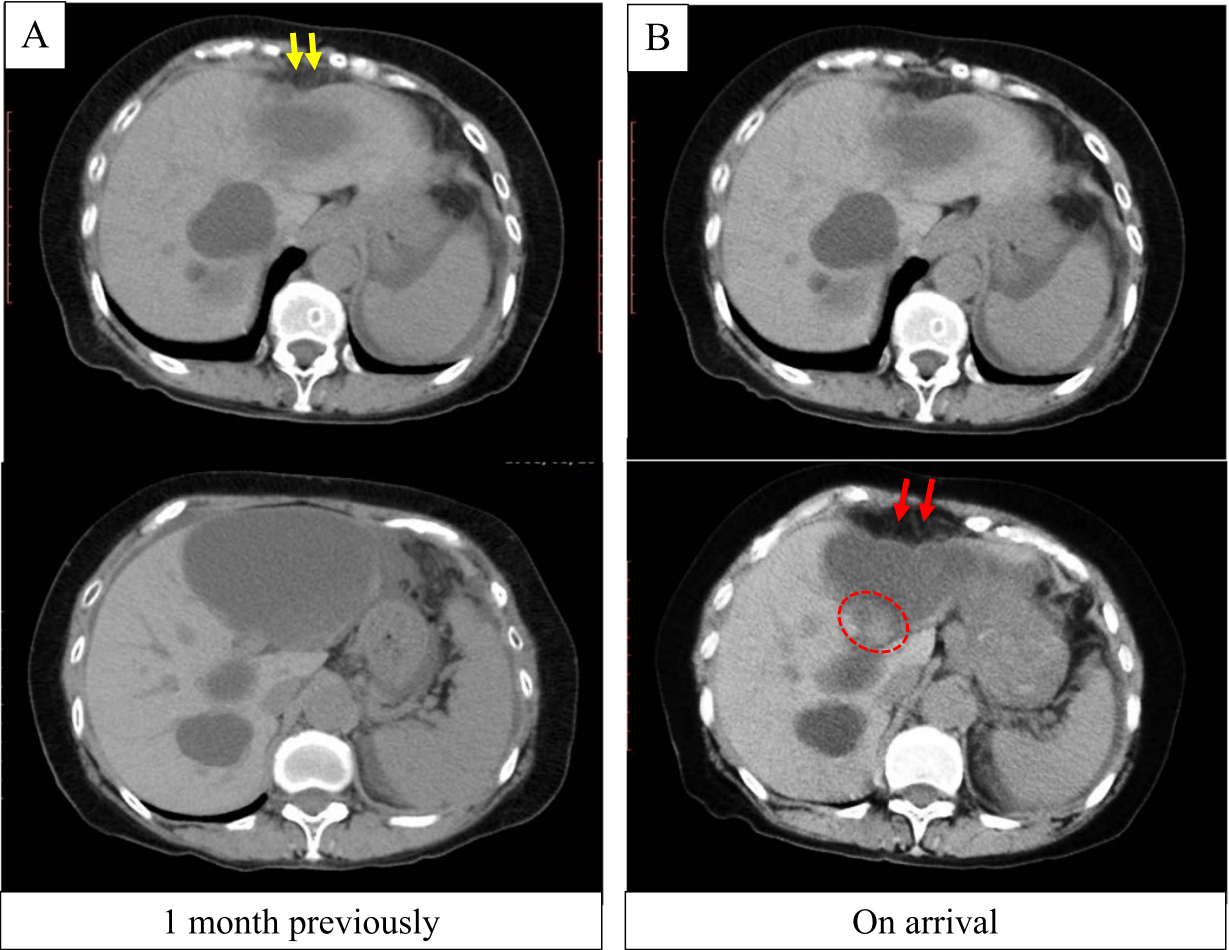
CT images of progression of hepatic cyst rupture. a CT image 1 month before presentation to our hospital. The largest cyst showed an irregularly shaped wall on the ventral side (*above*, *yellow arrows*). At that time, the caudal part of the cyst kept circular (*below*). Some ascitic fluid was found around the spleen. b CT image on arrival to our hospital. Volume of the irregularly shaped cyst had obviously decreased (*red arrows*) and was present within a relatively high dense lesion (*red circle*)
